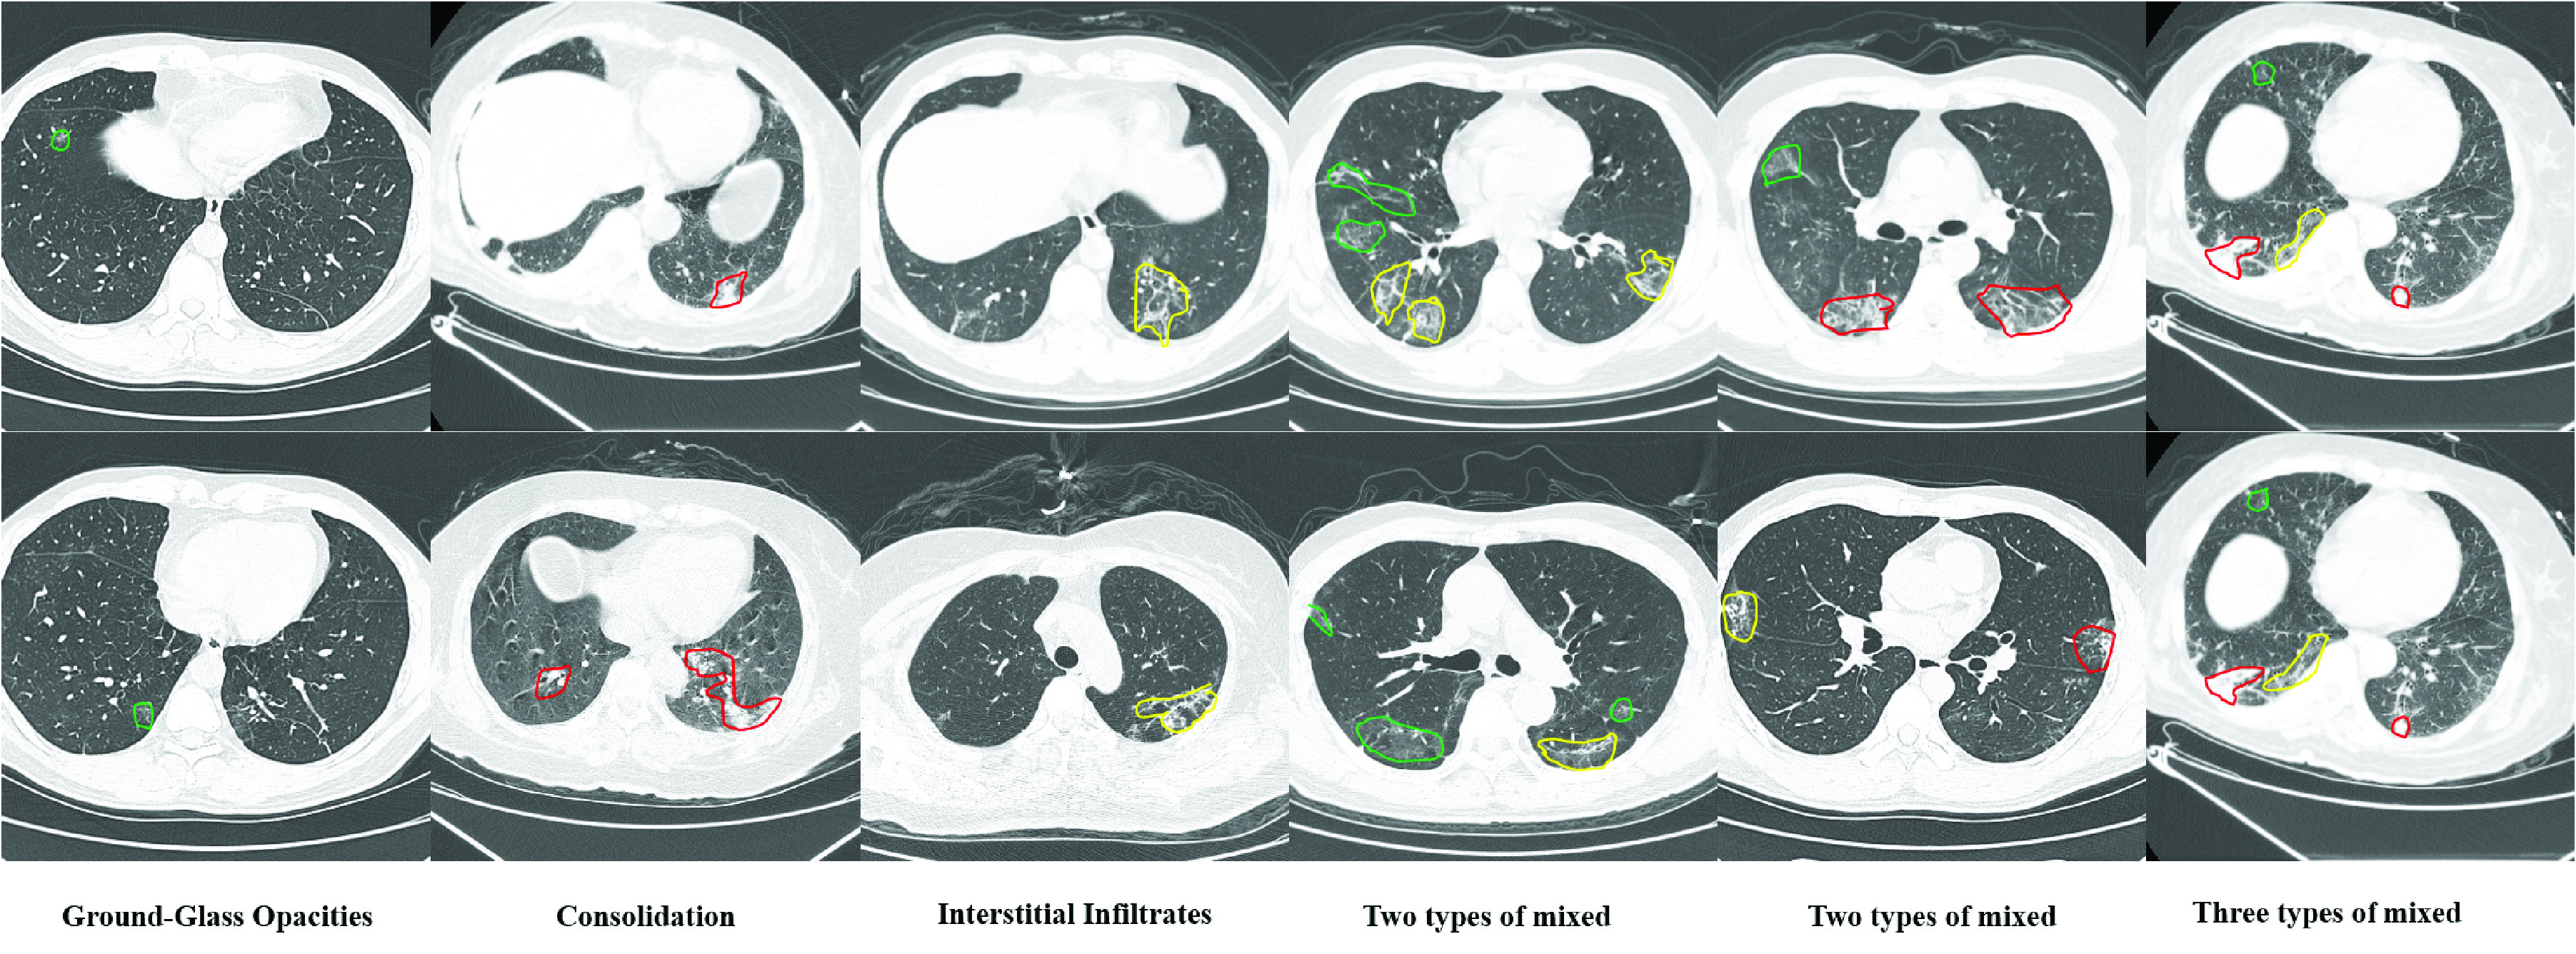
CT manifestations of different infection types. The samples in 1–3 column content single category on a CT slice. The samples in 4 and 5 columns include two categories. The last column shows the situation where the three types appear simultaneously. In the figure, green represents ground-glass opacities, yellow represents interstitial infiltrates and red represents consolidation.
